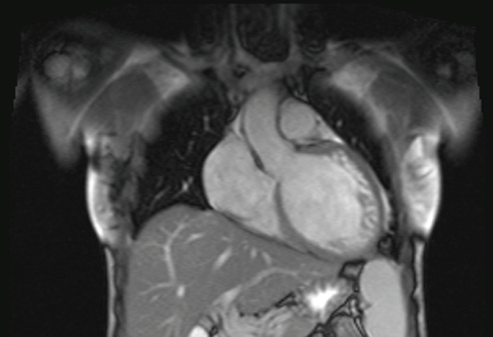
Visualization of trabeculae at MRI (frontal view)
